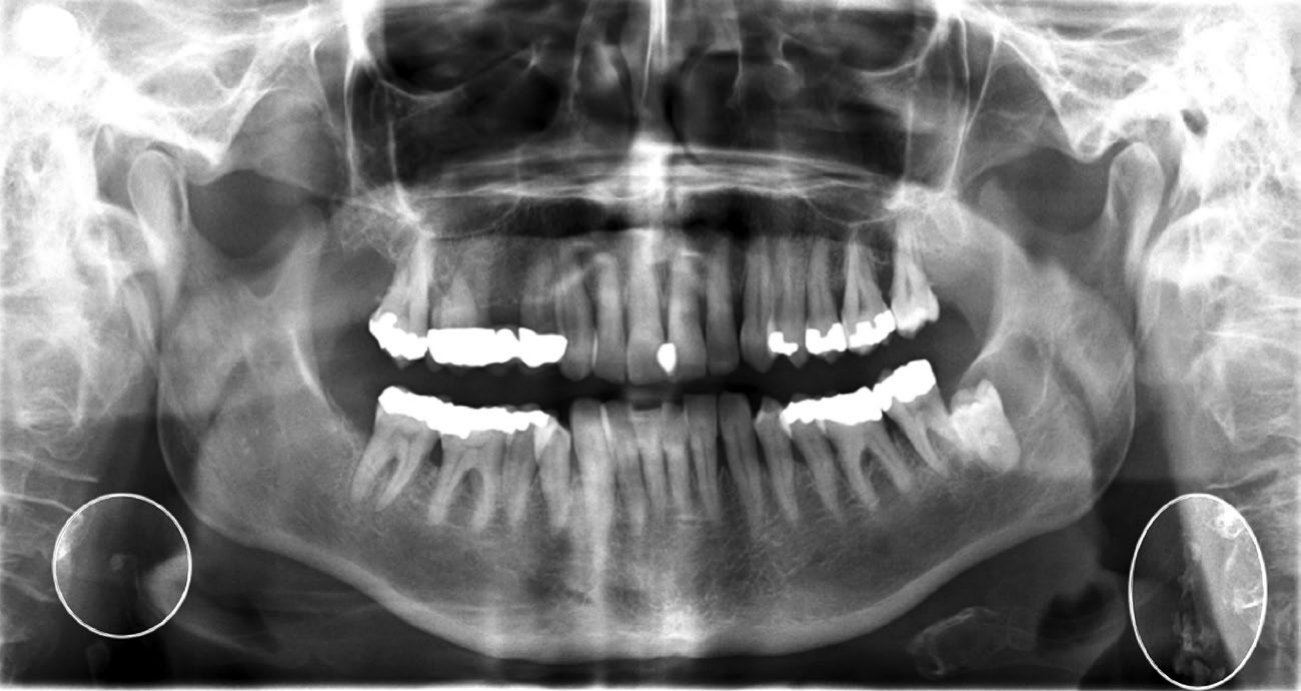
A panoramic radiograph depicting calcified carotid artery atheromas on the left and right sides
